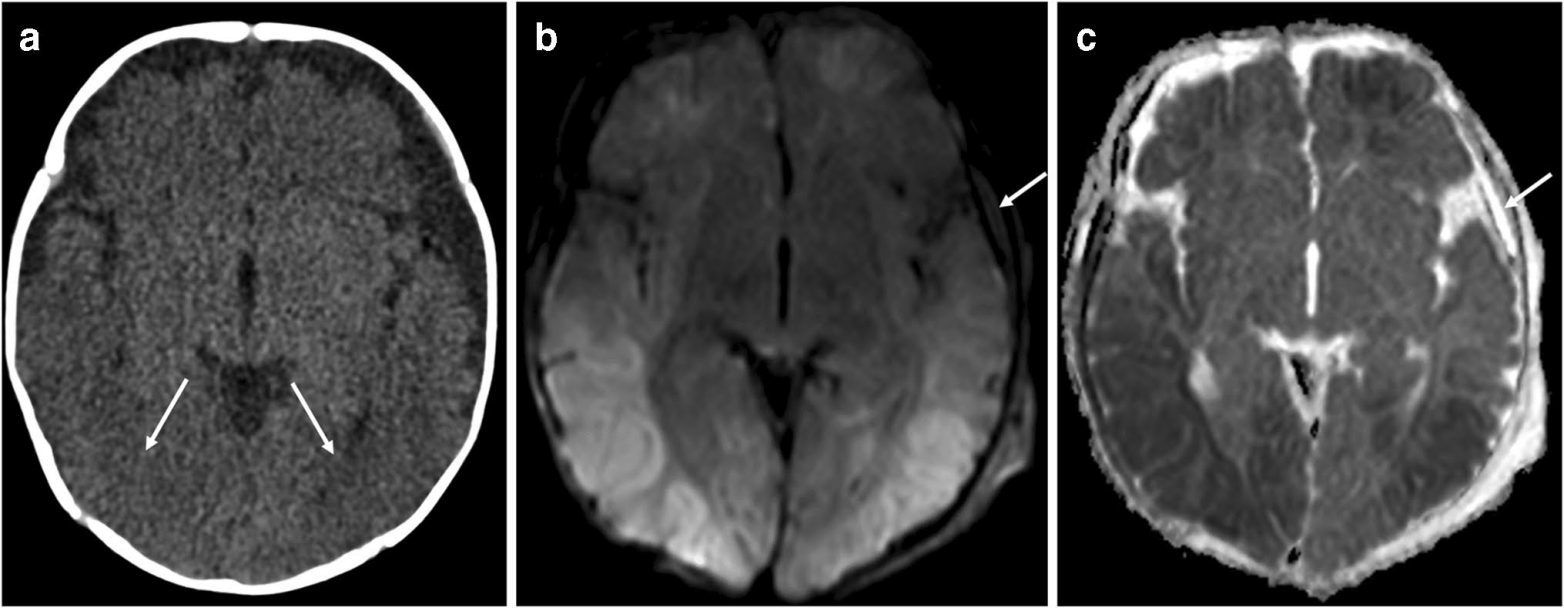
Selected cranial imaging in a 5-month-old girl. a The axial head CT (soft tissue window) demonstrates subtle predominately posterior (*arrows*) loss of grey-white matter differentiation. There is enlargement of the left extra-axial space without visible subdural haemorrhage. The axial MRI (b) diffusion-weighted imaging (DWI) and the (c) apparent diffusion coefficient (ADC) map, performed 12 h after (a), demonstrate corresponding recent diffuse hypoxic ischaemic injury in the same areas of grey-white matter differentiation loss visualised in (a) which are better depicted on MRI. An intermediate signal intensity small subdural collection (*arrow*) is visible in (b) with corresponding high signal intensity (*arrow*) in (c)
